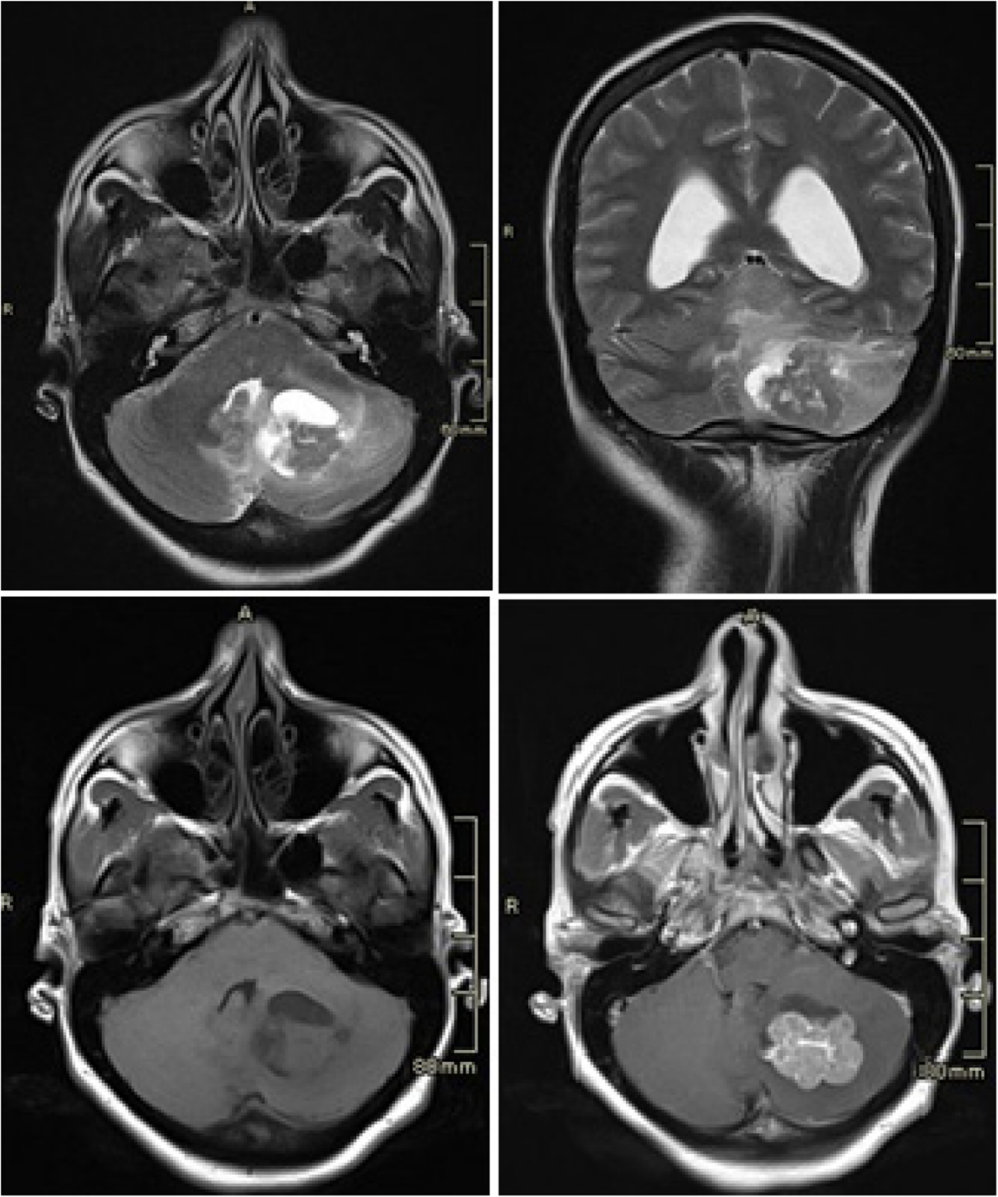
Brain magnetic resonance imaging. A nodular lesion with a cystic component of intra-axial location in the left cerebellar hemisphere is observed, whose enhancement component measures 23 × 31 mm (anteroposterior × transverse), is associated with vasogenic edema in the periphery and conditions the mass effect
